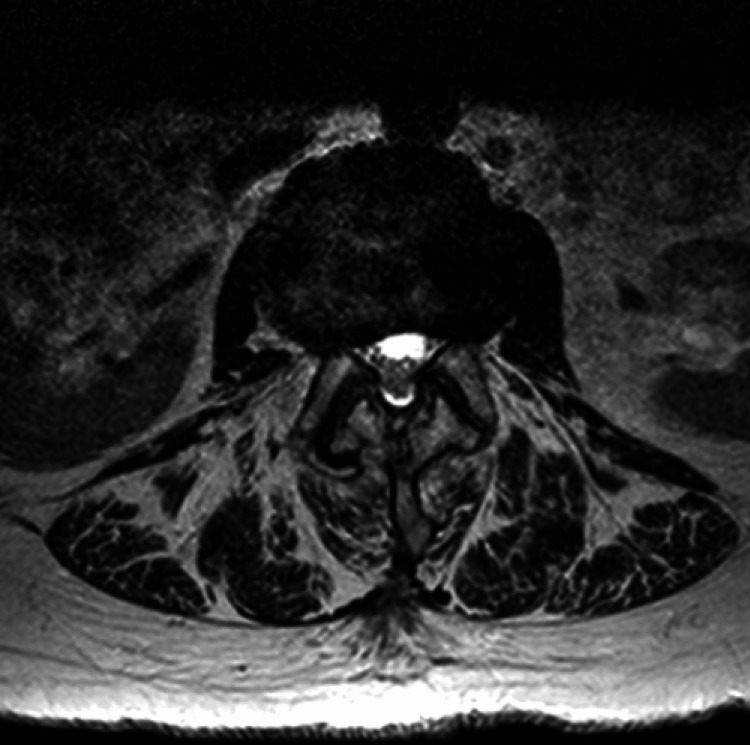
Axial MRI with L4-L5 facet joint space narrowing and osteophyte formation.
